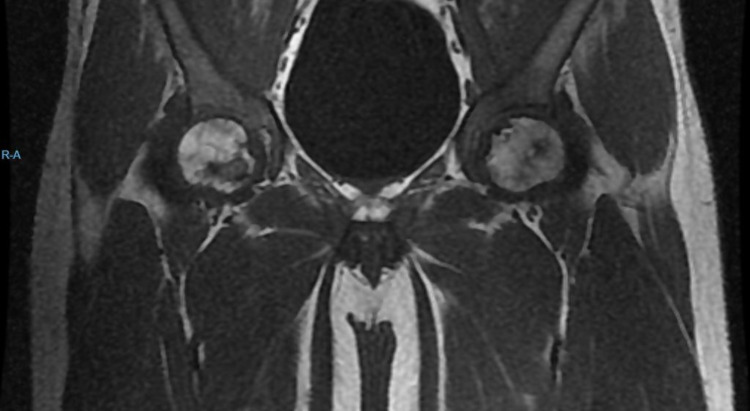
MRI of hip joint showing avascular necrosis of femur
